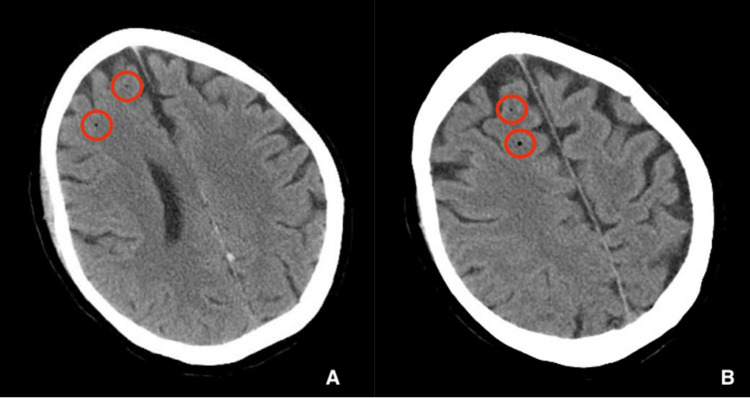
(A and B) CT head revealing two low-density foci (red circles) in two different CT sections of the right frontal lobe.
